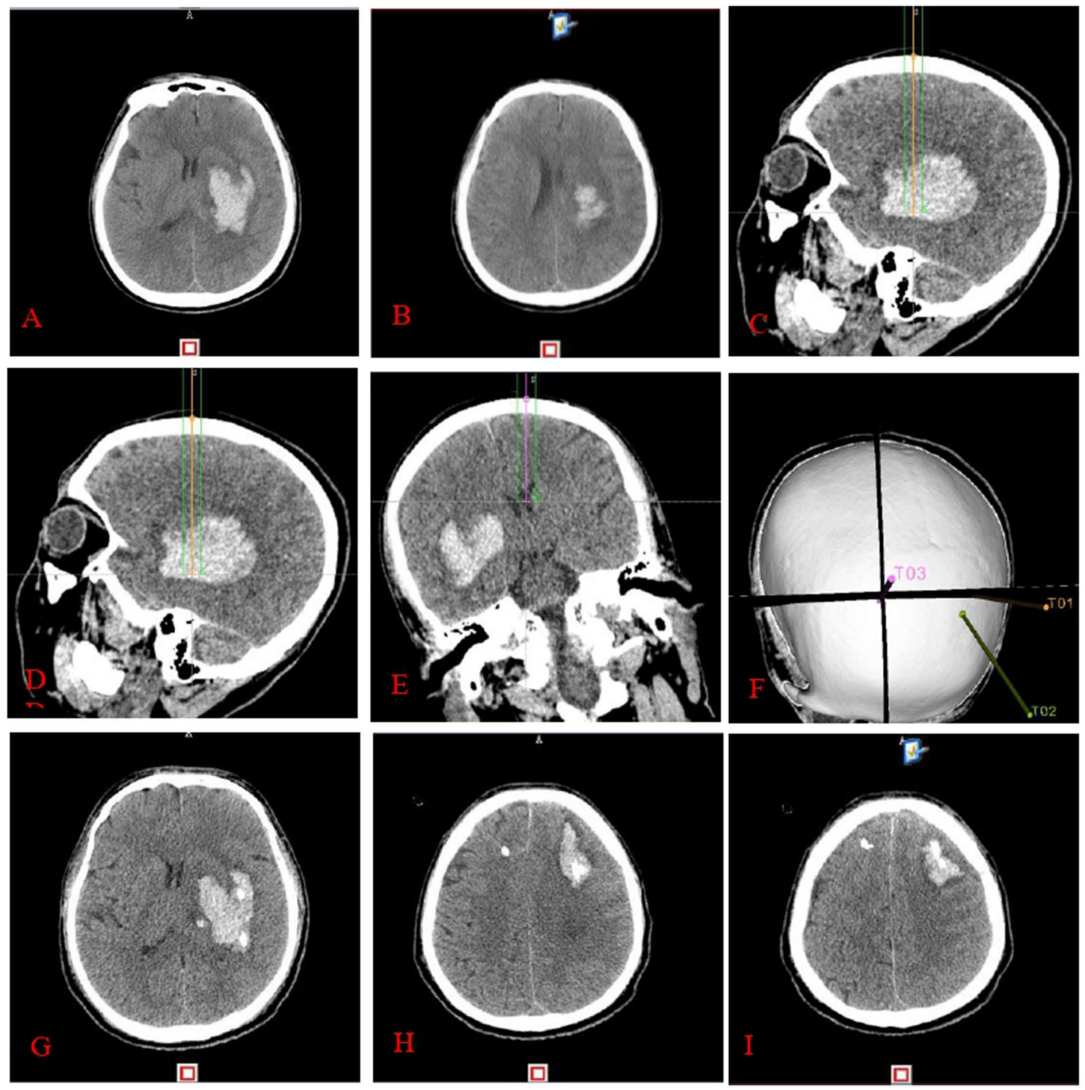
Completion of stereotactic surgery guided by cranial CT: preoperative cranial CT (A,B) reveals a left basal ganglia hematoma. The surgical route design (C–F) involved targeting the anterior midpoint (C), posterior midpoint (D), and anterior pedicle of the right ventricle (E) of the hematoma, with puncture placement and drainage performed by a neurosurgical robot. Three-dimensional reconstruction image of the puncture pathway (F). Cranial CT (G–I) performed within 24 h postoperatively reveals an enlarged hematoma and hematoma formation around the drainage tube.
